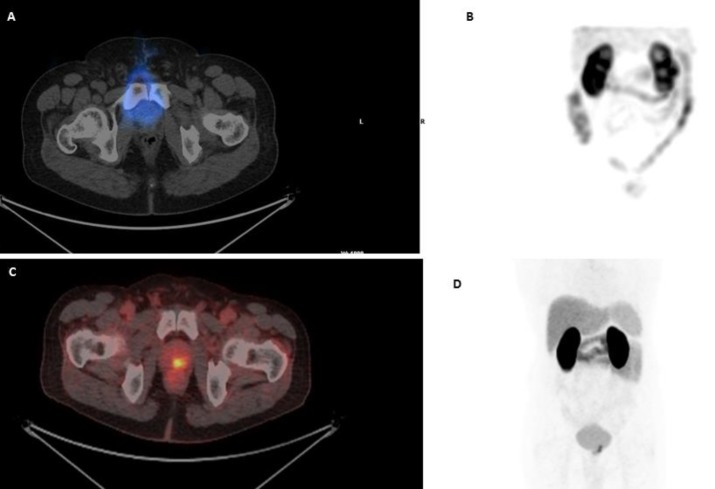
^99m^Tc-PSMA SPECT/CT transaxial view (A) of the pelvis and planar anterior view (B) shows no PSMA uptake in the prostate gland. ^68^Ga-PSMA PET/CT fused images (C) and whole body scan (D) shows focal small PSMA avidity in the prostate gland
